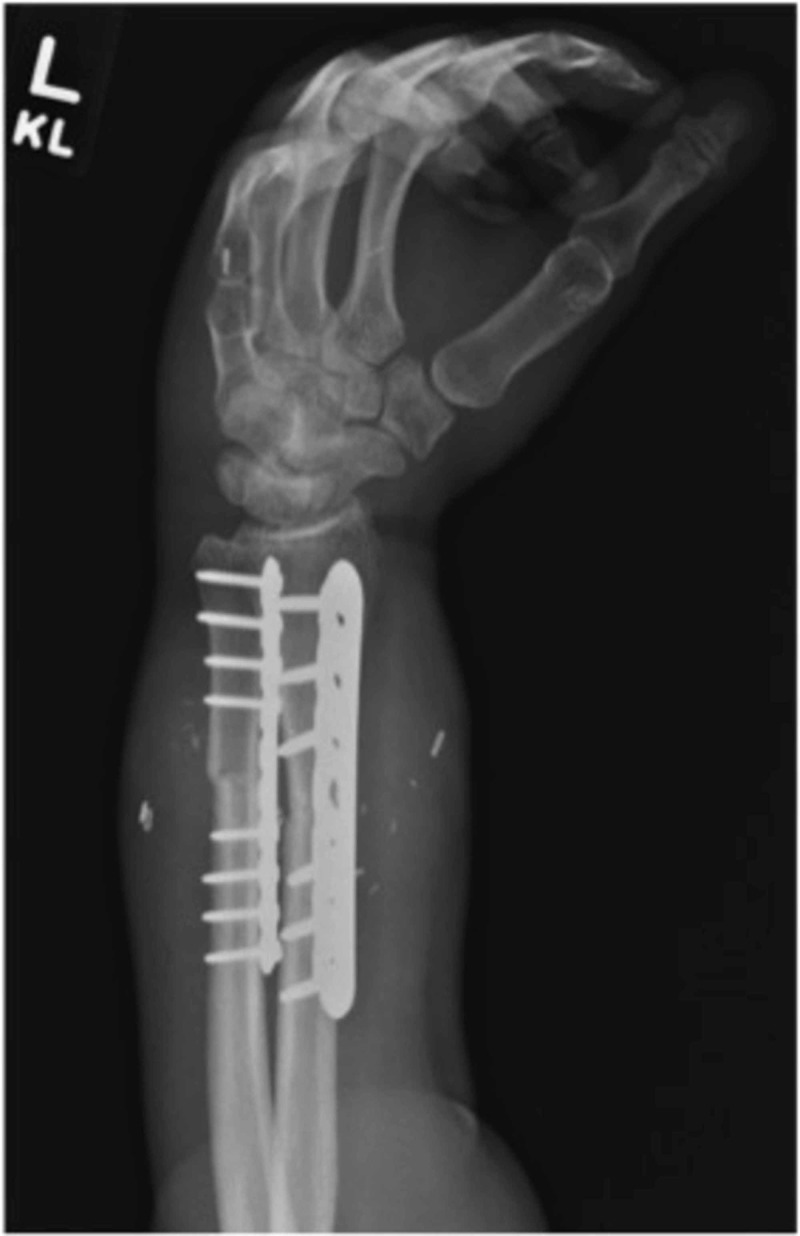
Oblique X-rays after plating Oblique X-rays demonstrating the radius and ulna forearm fixation after compression plating. Radius and ulna were both shortened by approximately 4 cm to allow for a tension-free repair of the vital soft tissues.
